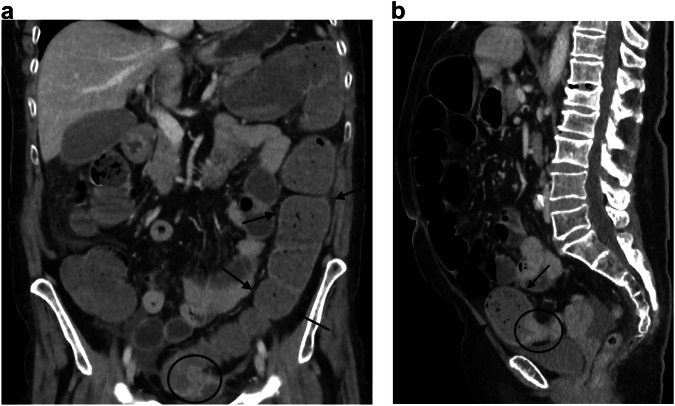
Large bowel obstruction caused by sigmoid cancer. Coronal and sagittal portal phase CT (a, b) in a 78-year-old woman shows a distension of the large bowel (arrows) above an asymmetric and short-segment thickening of the sigmoid wall (circle) narrowing the colonic lumen
